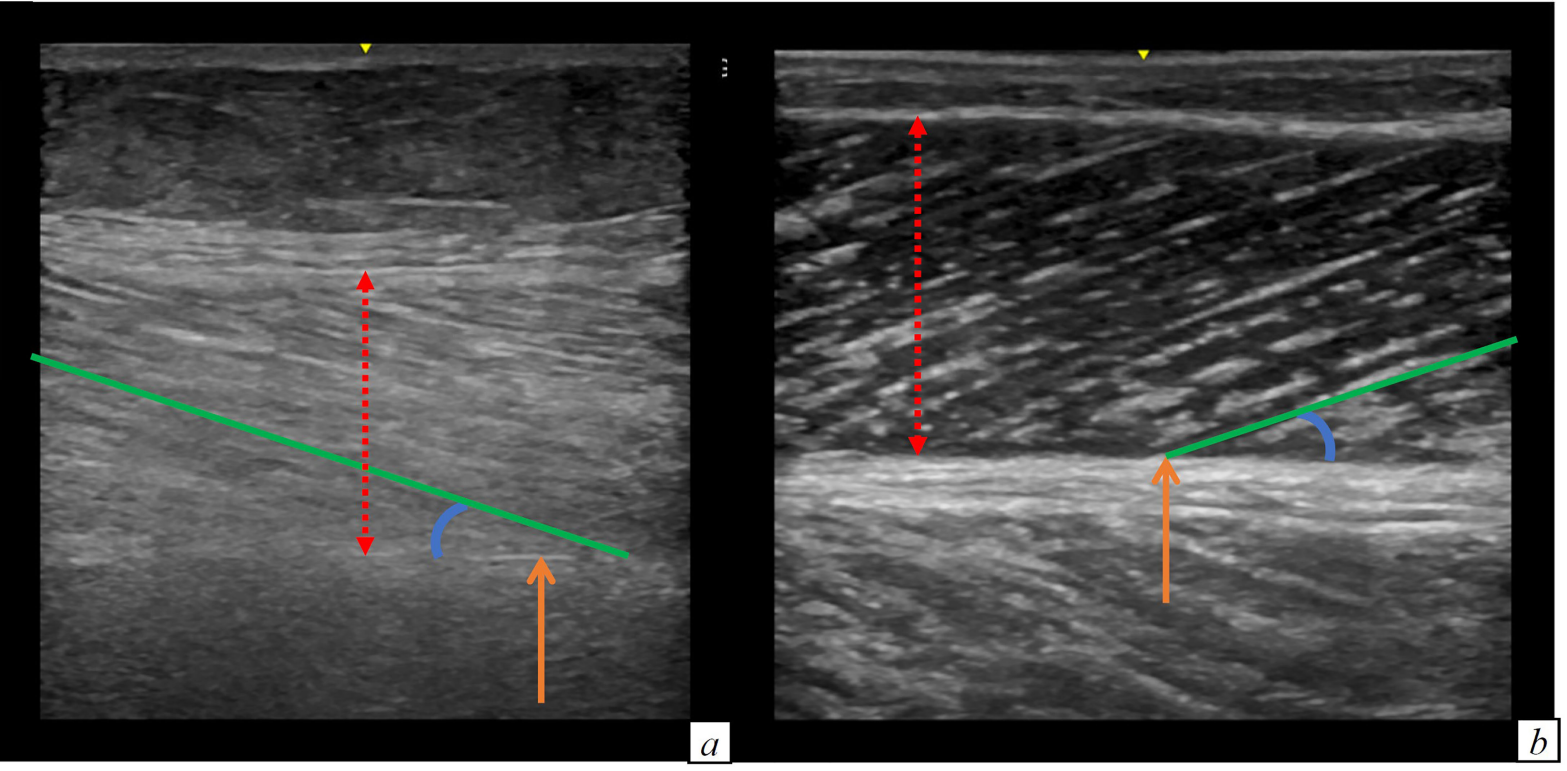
Comparison of the limb ultrasound images of a patient with (A) and without probable sarcopenia (B). (A) Muscle thickness: 1.54 cm, muscle stiffness 127 (B) Muscle thickness: 1.81 cm, muscle stiffness 46.8. RED DOTTED ARROW: muscle thickness; ORANGE ARROW: muscle deep aponeurosis; BLU CIRCLE LINE: pennation angle; GREEN line: muscle fascicle length.
